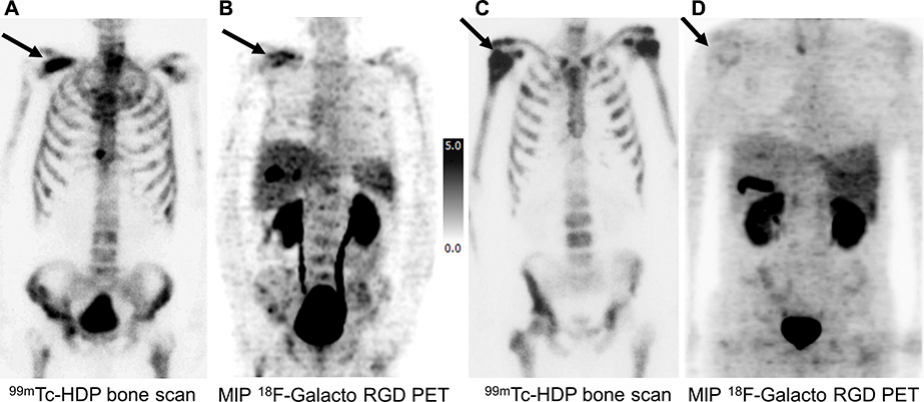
Imaging examples of a comparison of bone metastases in [^99m^Tc]HDP Bone Scan (A, C) and MIP [^18^F]Galacto-RGD PET (B, D) in two prostate cancer patients (A, B) 70 year old patient with bone metastases from prostate cancer under antiandrogen therapy and a PSA value of 55.26 ng/ml (initial staging cT3, cN0, cM1; Gleason score 8): [^99m^Tc]HDP Bone Scan (A) revealed an intense uptake in disseminated bone metastases (arrow indicating an osseous metastasis of the right scapula), which also showed a moderately to intensely elevated uptake in [^18^F]Galacto-RGD PET (B) in the majority of lesions. (C, D) 61 year old patient with bone metastases from prostate cancer under antiandrogen therapy and a PSA value of 175 ng/ml (initial staging: pT3a, pN0, cM0, G2; Gleason score 8): [^99m^Tc]HDP Bone Scan (C) revealed an intense uptake in disseminated bone metastases (arrow indicating a osseous metastasis of the right humerus). In [^18^F]Galacto-RGD PET (D), however, only faint or no uptake was seen in the bone metastases. These examples demonstrate, that despite very similar patterns of metastatic spread in bone scintigraphy, the findings in [^18^F]Galacto-RGD PET can be totally different. Note physiological uptake of [^18^F]Galacto-RGD in the liver, the spleen as well as in the gallbladder and in the urinary system.
